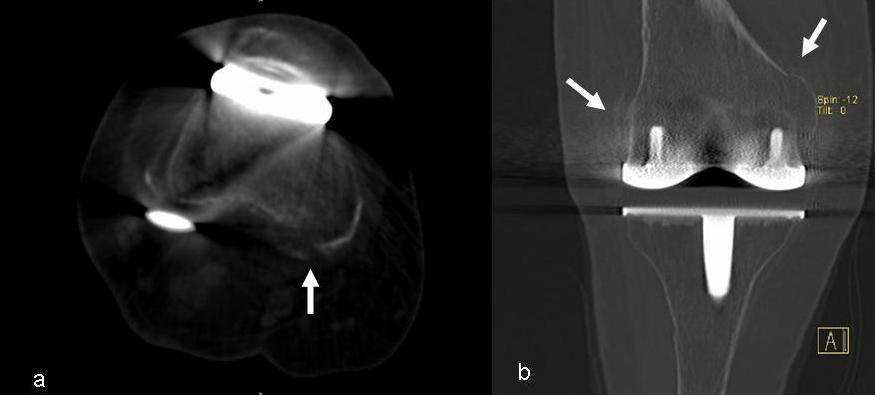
CT-scan of the right knee-joint. The fracture line of the periprosthetic fracture is marked by white arrows on an axial view (a) and coronar view (b).
